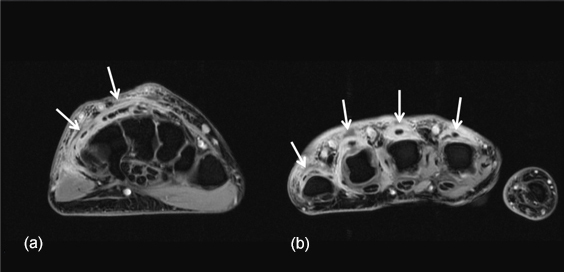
Axial post-contrast T1-weighted fat-suppressed images at the level
